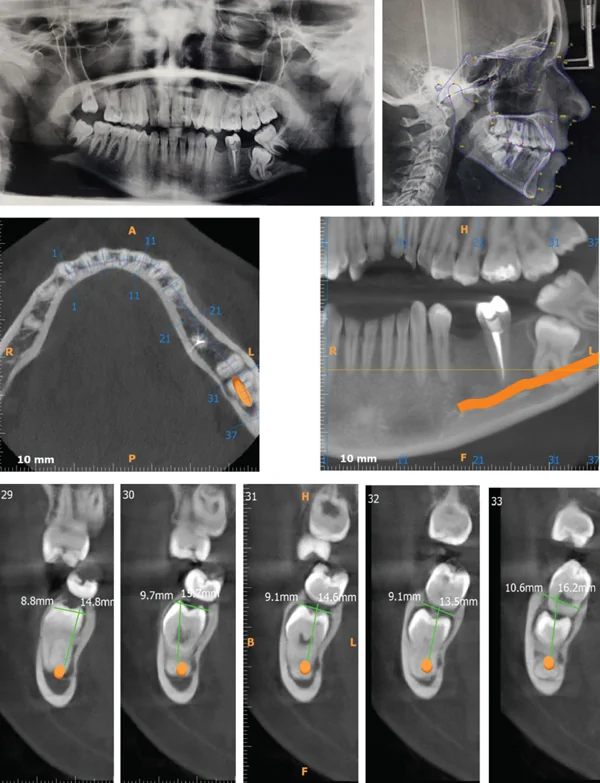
Initial panoramic radiograph, cephalogram, and CBCT.
